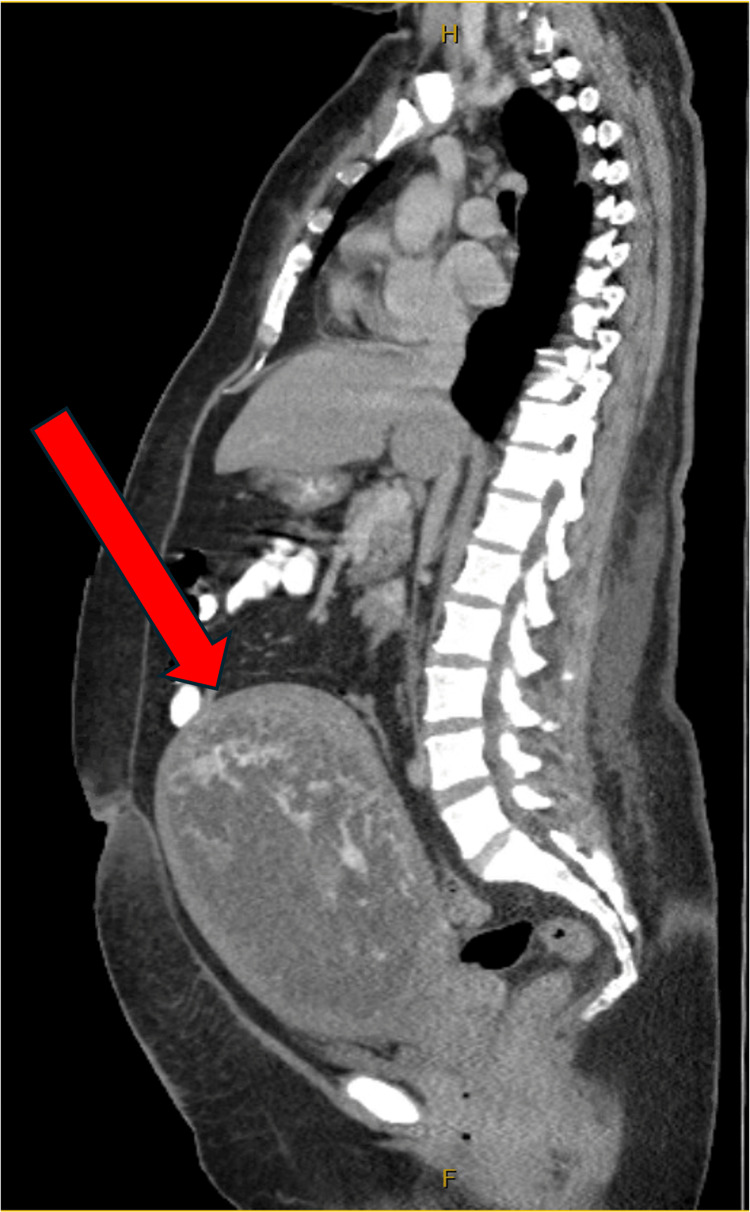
Computed tomography scan. The arrow points to the enlarged uterus. Computed tomography imaging reveals a markedly enlarged uterus above the level of the umbilicus. Hypodense intrauterine mass with heterogeneous reticular enhancements suggesting hydatidiform mole.
